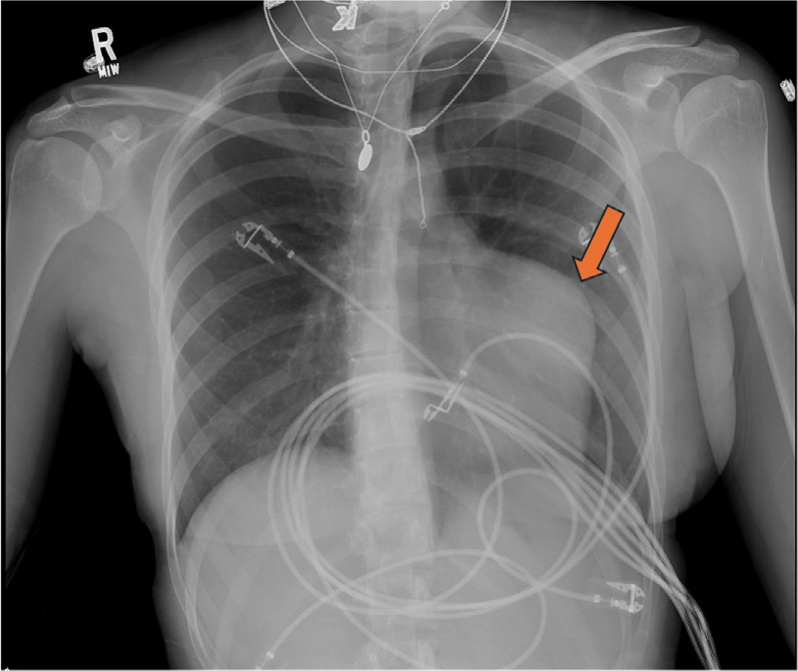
Chest X-ray Showing Abnormal Contour of Left Heart Border, Which Corresponds to Aneurysm of the Left Anterior Descending Artery Seen on Computed Tomography Angiography Chest
